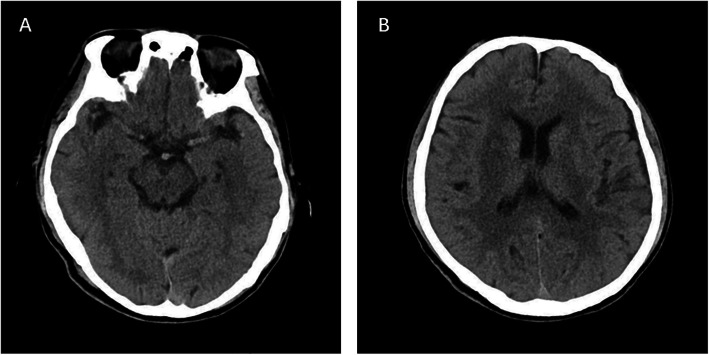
Brain CT images at different cross sections (a & b), which indicated lacunar cerebral infarction
